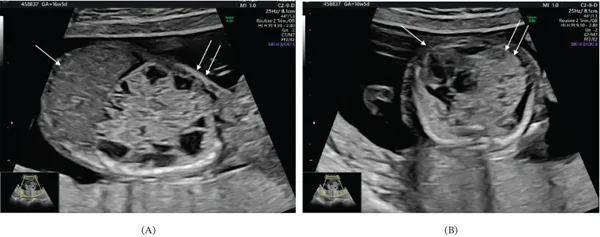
Ultrasound examination at 16 + 5 weeks. (A) A mass with mixed echogenicity and cystic appearance (double white lines). (B) The thoracic mass compressing the heart (single white line).
